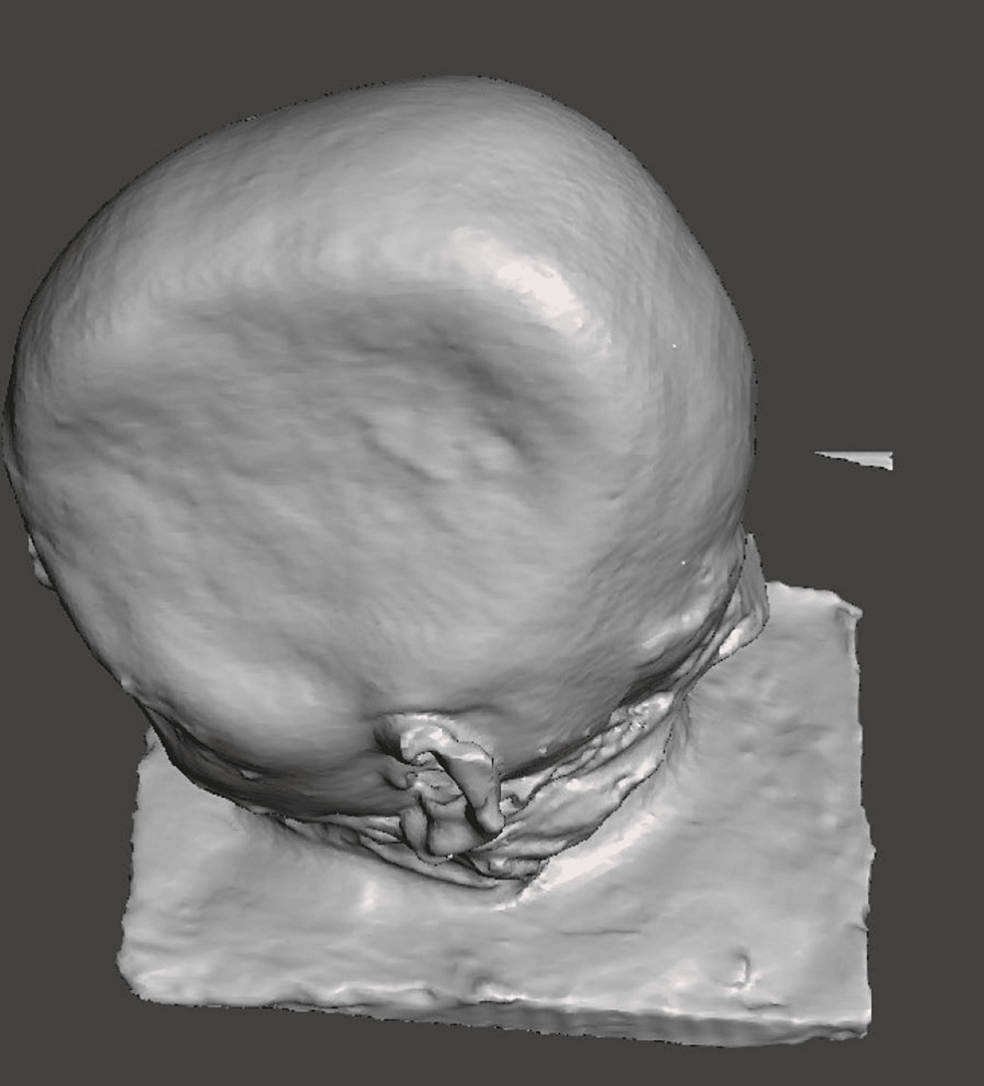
CT scan of the primary cast with cranial defect margins
